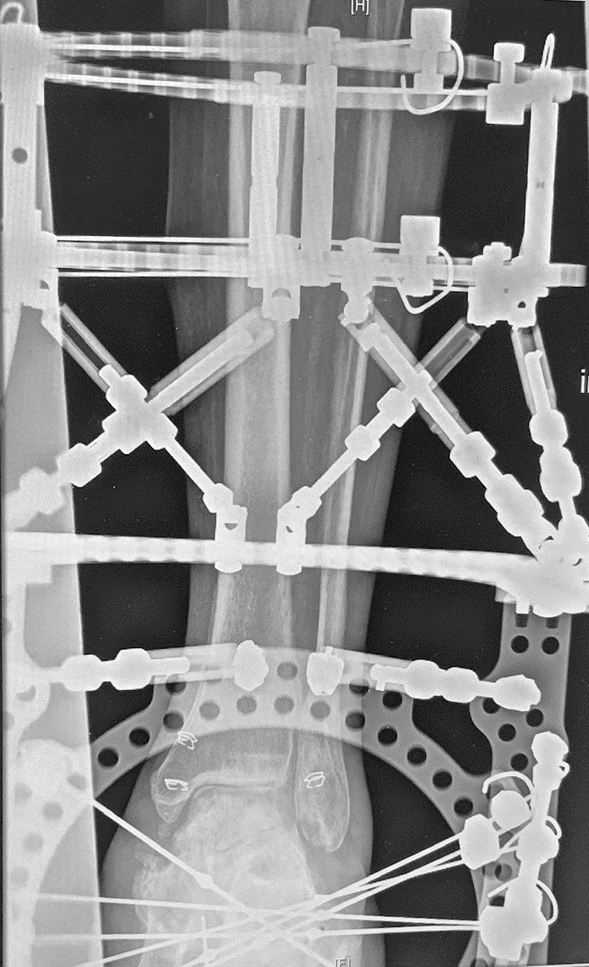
Anteroposterior x-ray view with attached TSF for simultaneous arthrodesis in tibiotalar und subtalar joints
